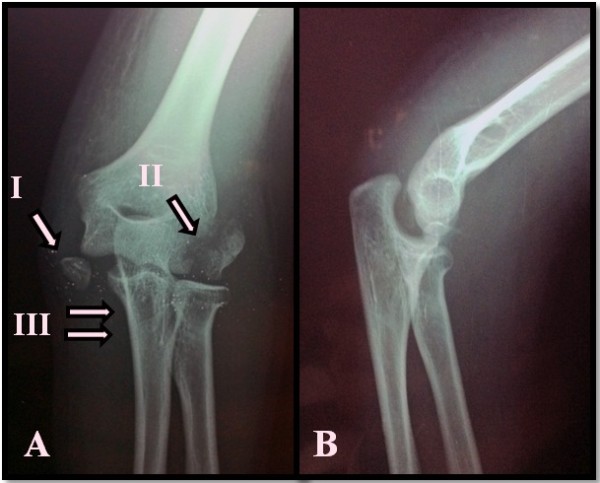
X-ray views of the left elbow. (A) Anteroposterior X-ray view of the left elbow (I: medial epicondyle avulsion fracture, II: lateral epicondyle displace fracture, III: humero-ulnar joint dislocation); (B) Lateral X-ray view of the left elbow shows widening of the joint space.
